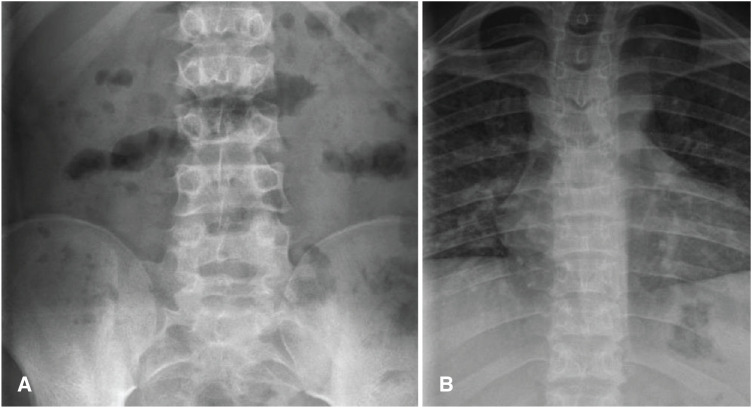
Bone X-ray findings in a patient with Schimke Immunoosseous dysplasia. (A) Lumbar spine and (B) Thoracis spine showing relatively flattened vertebral bodies with irregularly outlined endplates.
